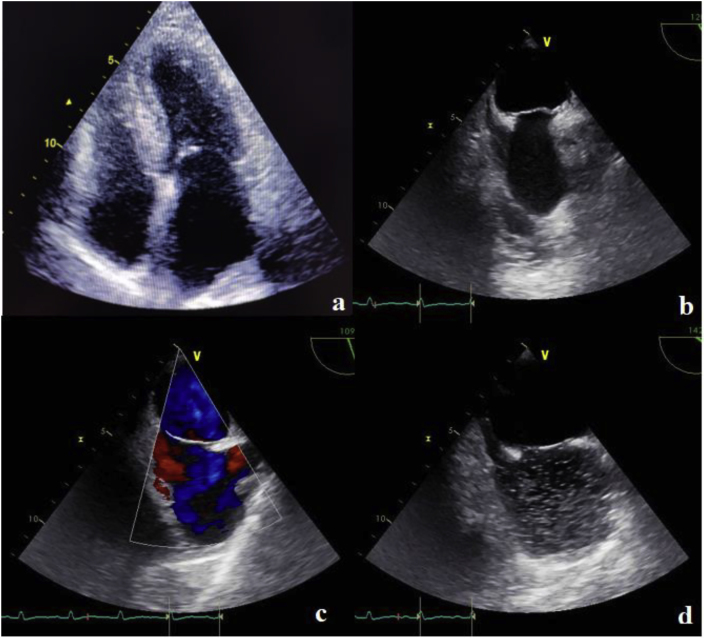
Six weeks Follow-up TTE apical four-chamber view (a) showing a normalization of the right heart cavities completed by a TEE (b) showing no septal aneurysm, ASD, nor a PFO. No right-to-left shunt on doppler flow (c) as well as a negative bubble test (d).
